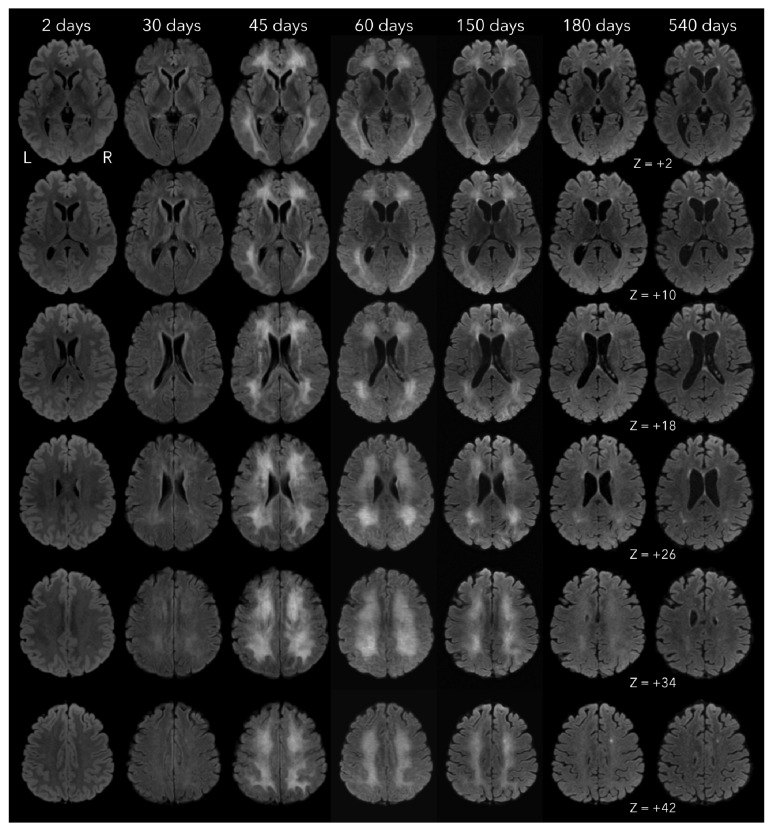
Comparison of MRI scans across different time-points after exposition. 30 and 45 days after initial event, FLAIR sequences show a diffuse and confluent hyperintensity involving predominantly the periventricular and deep white matter. Follow-up MRI scans (60, 150, 180, and 540 days after initial event) show gradual regression of the FLAIR hyperintensity.
